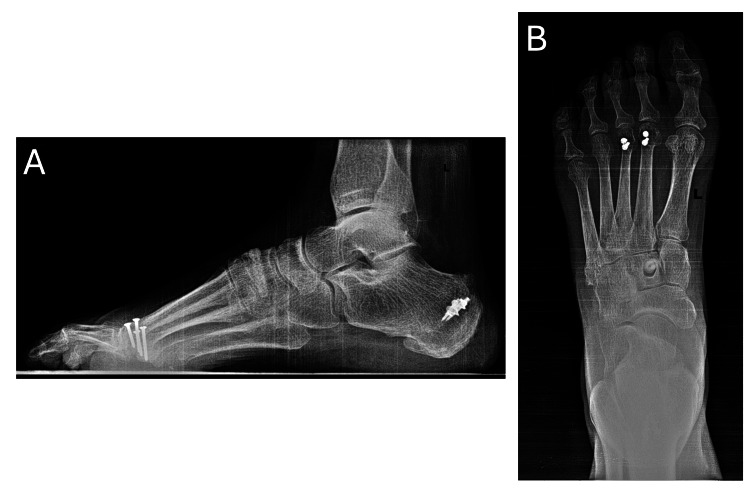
Final postoperative radiographs of the left foot Postoperative AP and lateral radiographs, October 2025. (A) Lateral view showing the interference screw in the intermediate cuneiform, hardware in the second and third metatarsal heads, and bone anchors in the posterior calcaneus. (B) AP weight-bearing radiograph demonstrating a normalized metatarsal parabola and the interference screw within the intermediate cuneiform.
